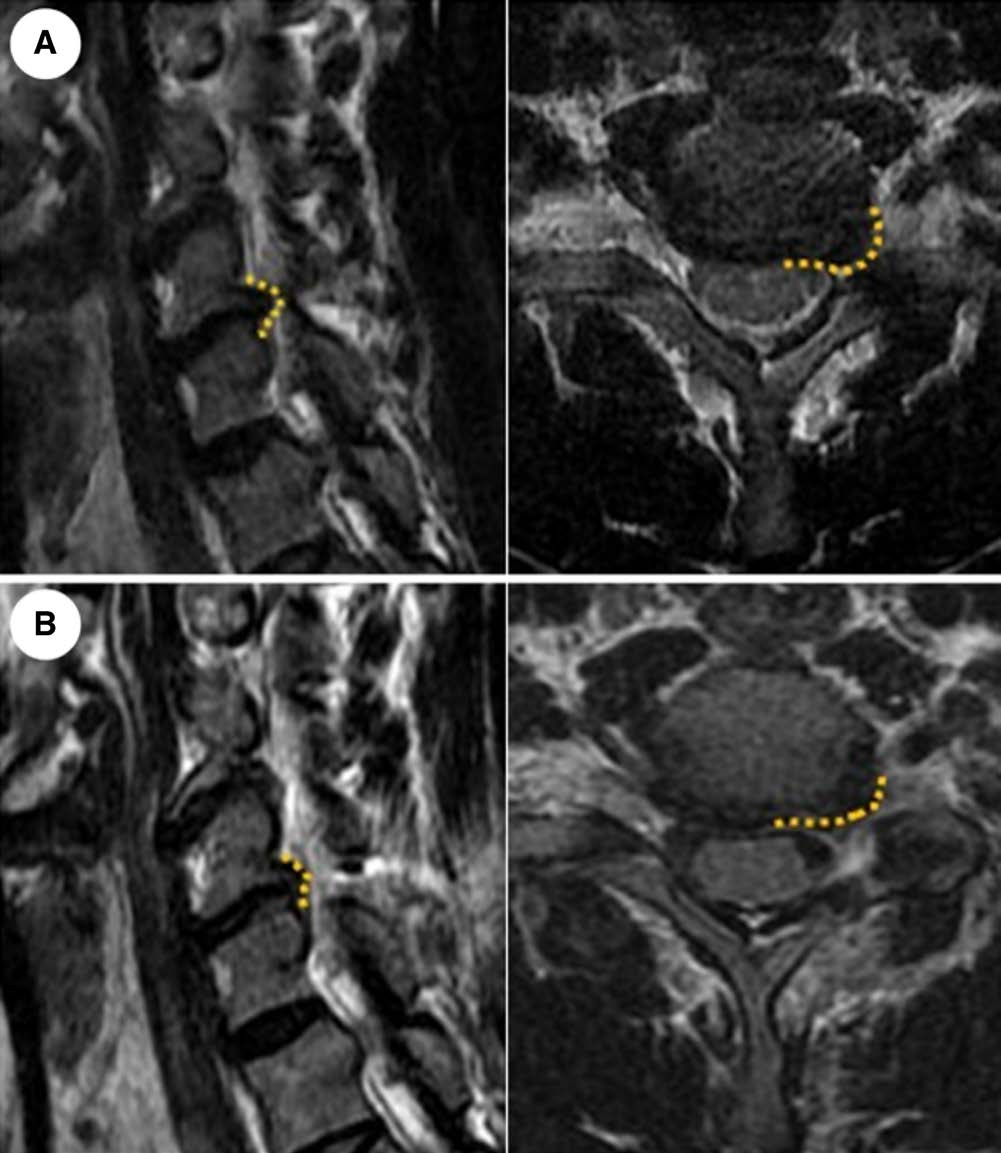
Preoperative and postoperative images. Preoperative MRIs show C6/7 disk protrusion on the left subarticular zone, and the same levels of 1 d postoperative images show a relatively widened space on the zone and removed herniated disk with partial laminectomy and facetectomy. The yellow dashed lines present the annular outline of the target disk. MRI = magnetic resonance imaging.
